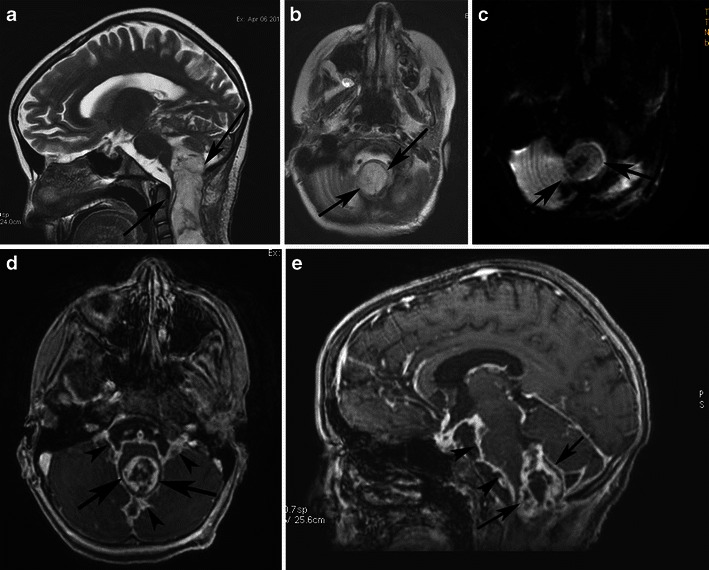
A pilocytic astrocytoma extending to the upper cervical spine with dissemination after partial resection. a Sagittal T2-weighted and b axial T2-weighted images at the level of the foramen magnum show a high-signal cyst-like mass (arrows). c The lesion is hypointense on diffusion-weighted imaging (arrows). Contrast-enhanced axial (d) and sagittal T1-weighted (e) images of the brain show inhomogeneous enhancement of the mass (arrows), as well as diffuse enhancement of the basilar cisternal spaces (arrowheads)
